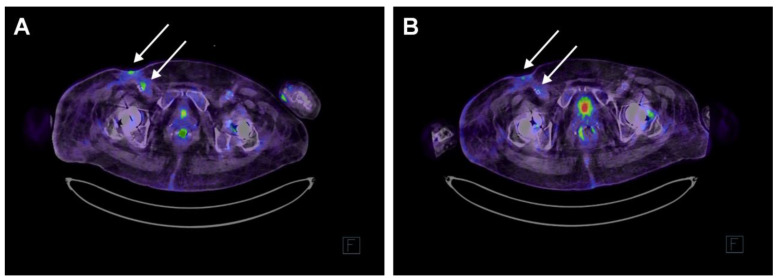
Axial fused PET-CT image at level of inguinal region. (A) Two weeks prior to the phage treatment. In the right groin, next to the a. iliaca externa stent, there was minor FDG (fluorodeoxyglucose) uptake SUVmax (maximum standardized uptake value) 4.9. On the surface of the skin of the right groin, there was a slightly higher uptake SUVmax 8.1. (B) PET-CT scan two weeks after the phage therapy course. In the area of the vascular stent in the right groin/femoral region, there was no residual activity to be mentioned. In the right groin, there was minor uptake superficially in the skin/scar area SUVmax 3.8. Arrows pinpoint the locations with altered metabolic activities. As the marker of glucose metabolism, 18-FDG 244 MBq was used, and imaging was performed with Siemens Biograph Vision.
